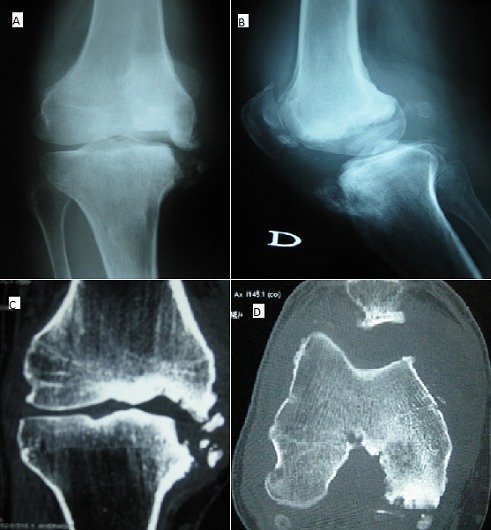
(A, B) radiography of the right knee (face and profile incidence): destruction of the internal femoral condyle with osteolysis of the internal tibial plateau associated with multiple bone constructions; (C, D) knee scan in sagittal and axial section: destruction of the internal femoral condyle with osteolysis of the medial border of the internal tibial plateau associated with multiple bone constructions with the presence of intra-articular fragments, intra-articular effusion and thickening of the synovium
